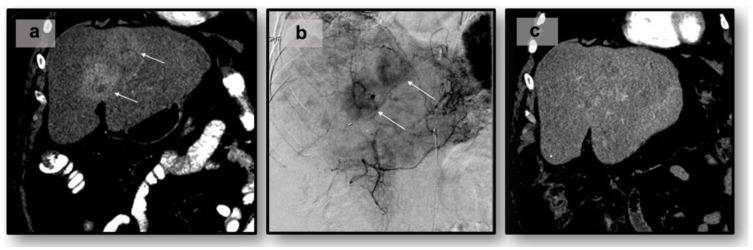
Transarterial chemoembolization of 55-year-old with liver-dominant metastatic NET of small bowel origin to the liver: (a) pre-procedure CT. White arrows indicate lesion(s): (b) intraprocedural angiogram, (c) post-procedural CT.
